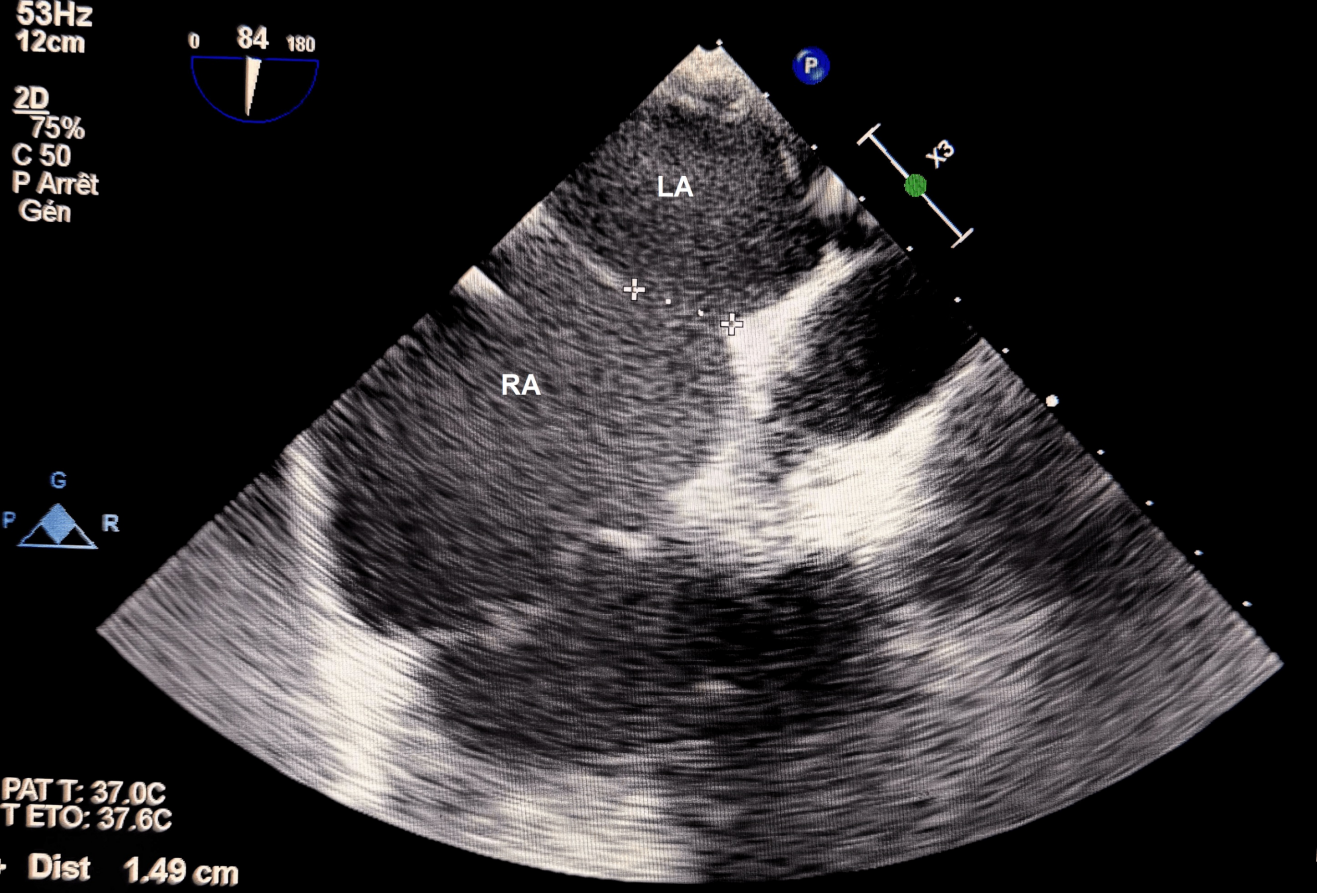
Transesophageal view showing the atrial septal defect (ASD) diameter RA: right atrium; LA: left atrium
